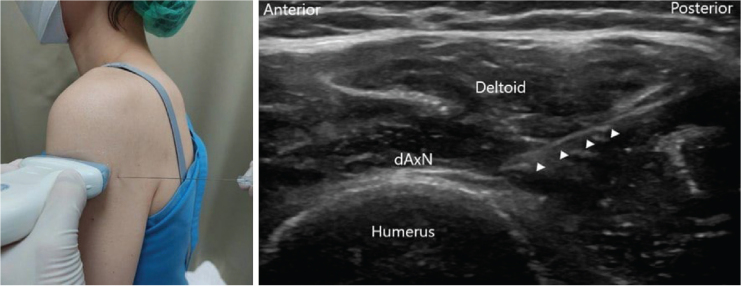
Ultrasound-guided injection at the superior lateral humeral level (dAxN) white arrowhead, needle; dAxN, distal part of the axillary nerve.
